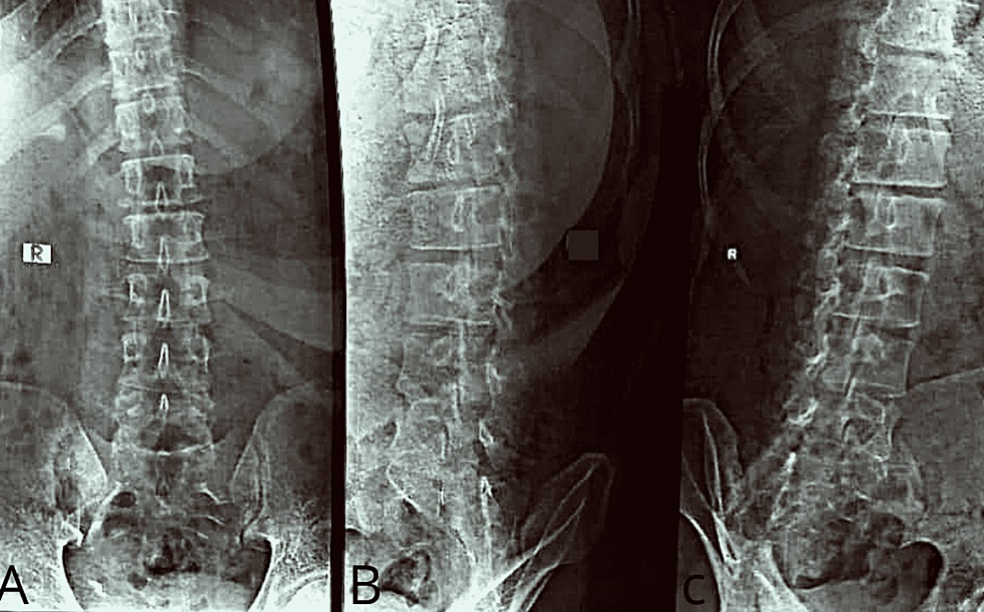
X-ray of the lumbo-sacral spine (A) antero-posterior view; (B, C) lateral view.
